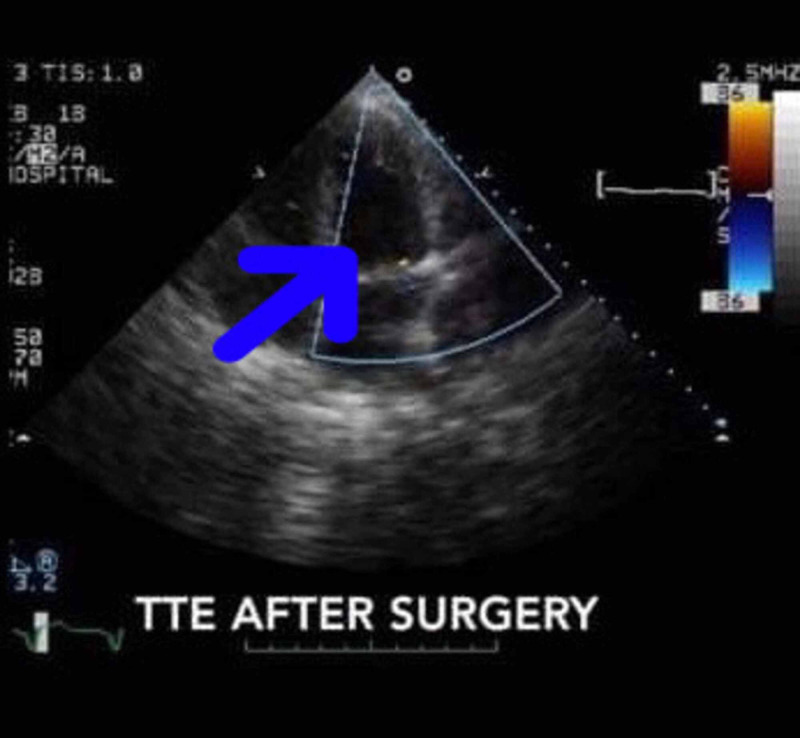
TTE after surgery TTE, transthoracic echocardiogram
